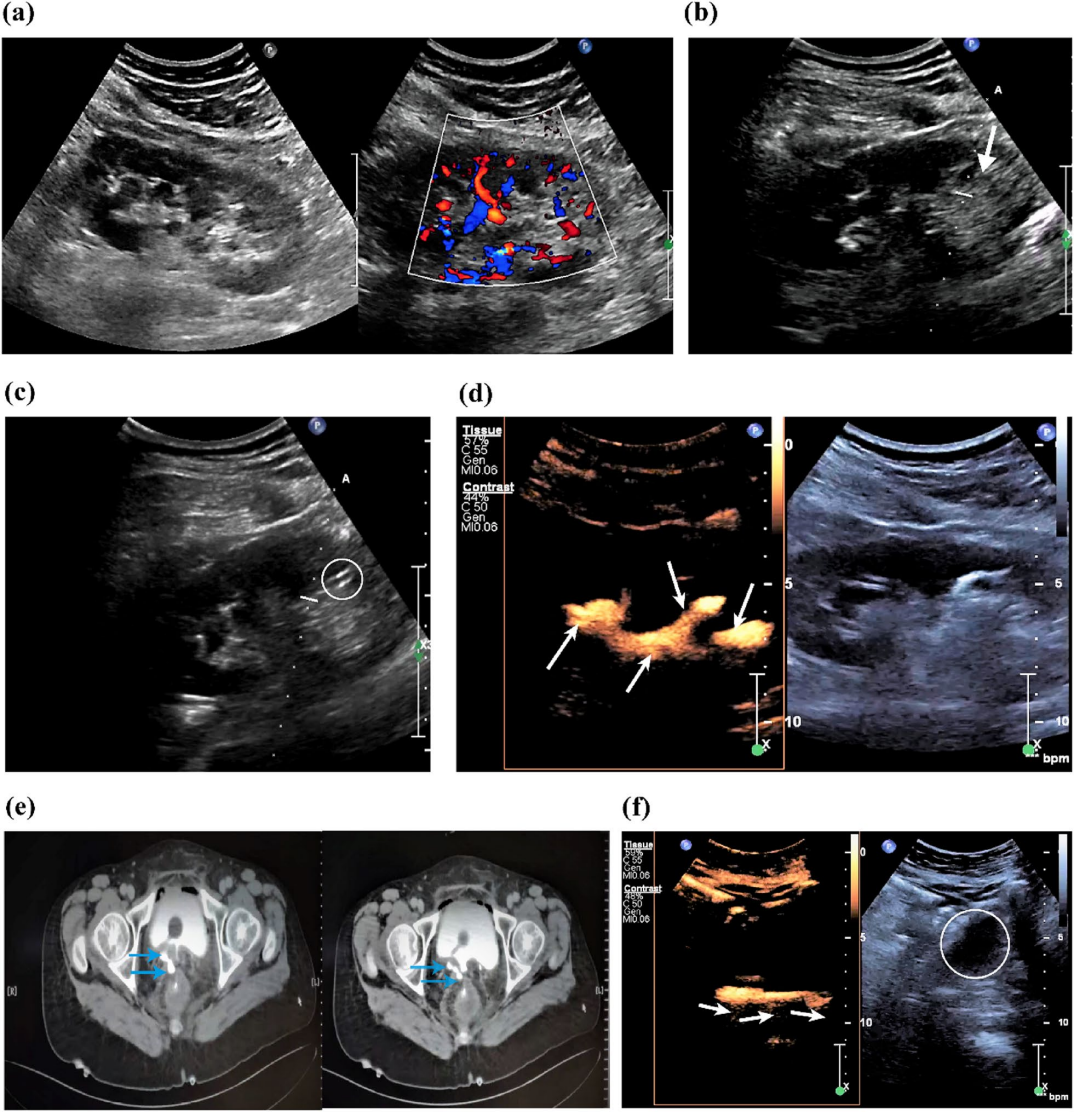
Images of a 53 year-old woman, diagnosed with ureteral fistula, underwent UCA assisted US guided PCN. a Conventional US and Doppler US demonstrate that none hydronephrosis occurs in renal system. b The course (arrow) of PTC puncture needle insertion. c The needle tip has entered the destination renal papilla (circle). d Withdraw the PTC needle, the renal calyces and its pelvis structures can be clearly observed via the diluted UCA solution be injected (arrowheads). e CT results manifested that the right ureter near the bladder opening was unclear and a little contrast medium was visible in the pelvis (arrowheads). f UCA media only presents in the patient's ureter (arrow) and not in the bladder (circle), suggesting ureteral fistula occurred
